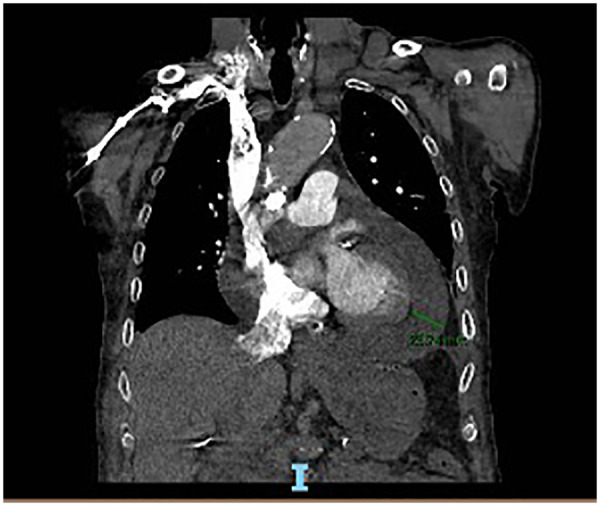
Coronal CT scan of the chest displaying the thoracic cavity, including lungs, heart, and adjacent structures, with significant white areas indicating abnormal tissue or mass along the mediastinum and left lung region.
